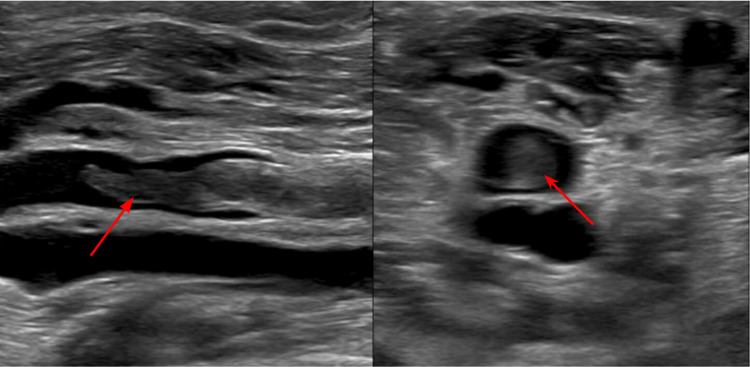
Ultrasound of the lower extremity showing thrombus in the right distal popliteal vein (arrow) in different views of ultrasound.
